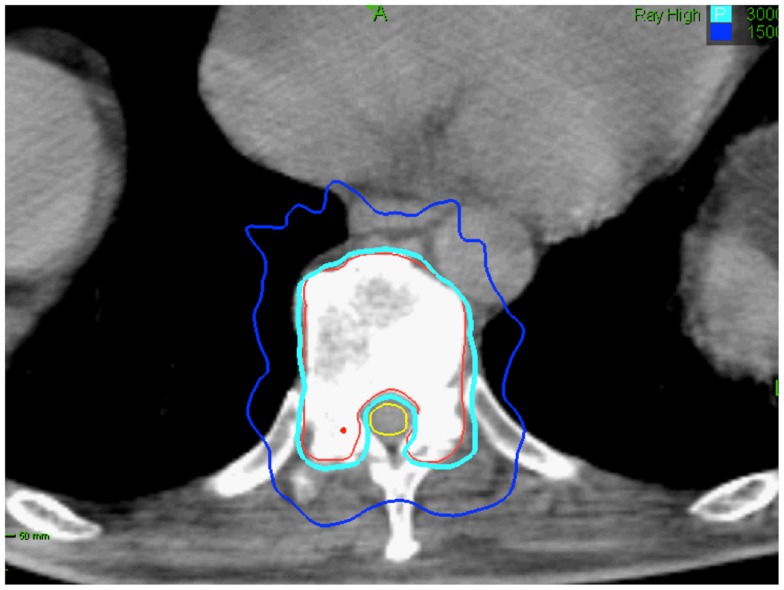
Seventy-five-year-old gentleman with oligometastatic prostate cancer and a T11 vertebral body metastasis. The decision was to proceed with ADT and SBRT. ADT was initiated. The vertebral body was treated with 30 Gy in 5 fractions. Treatment planning axial computed tomography images demonstrating the GTV (red) and spinal cord (yellow). Isodose lines shown as follows: 100% of the prescription dose (light blue line) and 50% of the prescription dose (dark blue line). The maximum point to the spinal cord and esophagus were 30 and 35 Gy, respectively (69).
